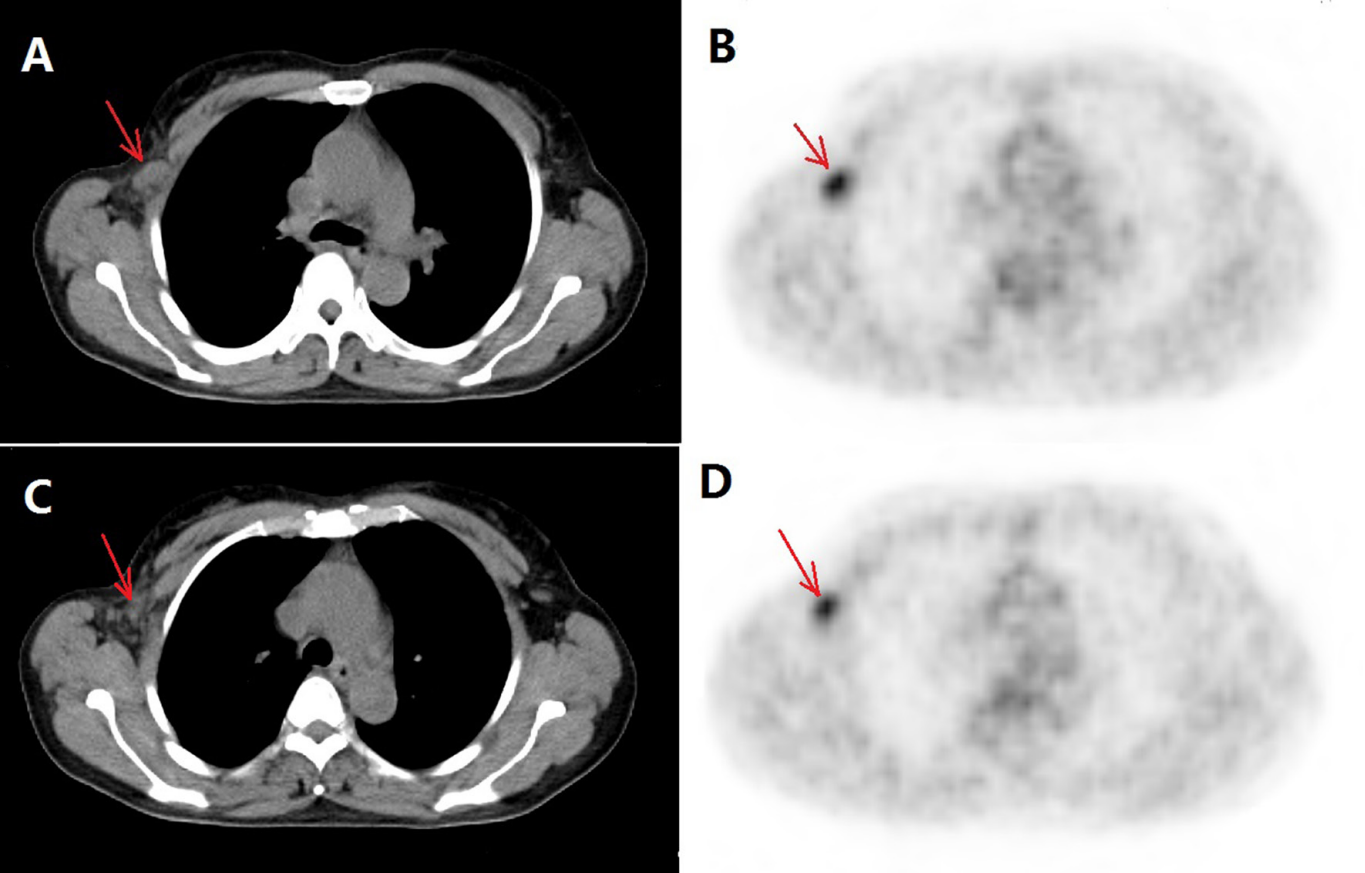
A false negative case involving a T2N1 breast primary lesion (HER2-positive) and right axillary lymph node metastasis before and after neoadjuvant chemotherapy (NAC) plus trastuzumab Transverse slices of CT and PET images show that the size of the right axillary lymph node (ALN) metastasis is 2.3 × 1.3 cm A. with high FDG uptake and a baseline SUVpeak of 5.16 B. After NAC plus trastuzumab treatment, the right ALN shrank C.; FDG uptake decreased and the SUVpeak was 2.72 D. The ΔSUVpeak is − 47% (from 5.16 to 2.72), less than the threshold of − 80%, but the result of ALN dissection was pathological complete response. ΔSUVpeak refers to the difference in standard uptake values between baseline and after two NAC cycles.
